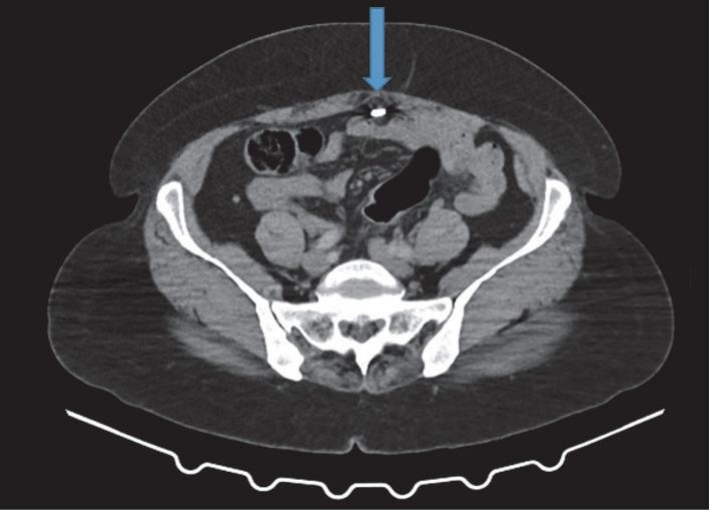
CT scan in axial view revealed a surgical blade in the abdomen.
